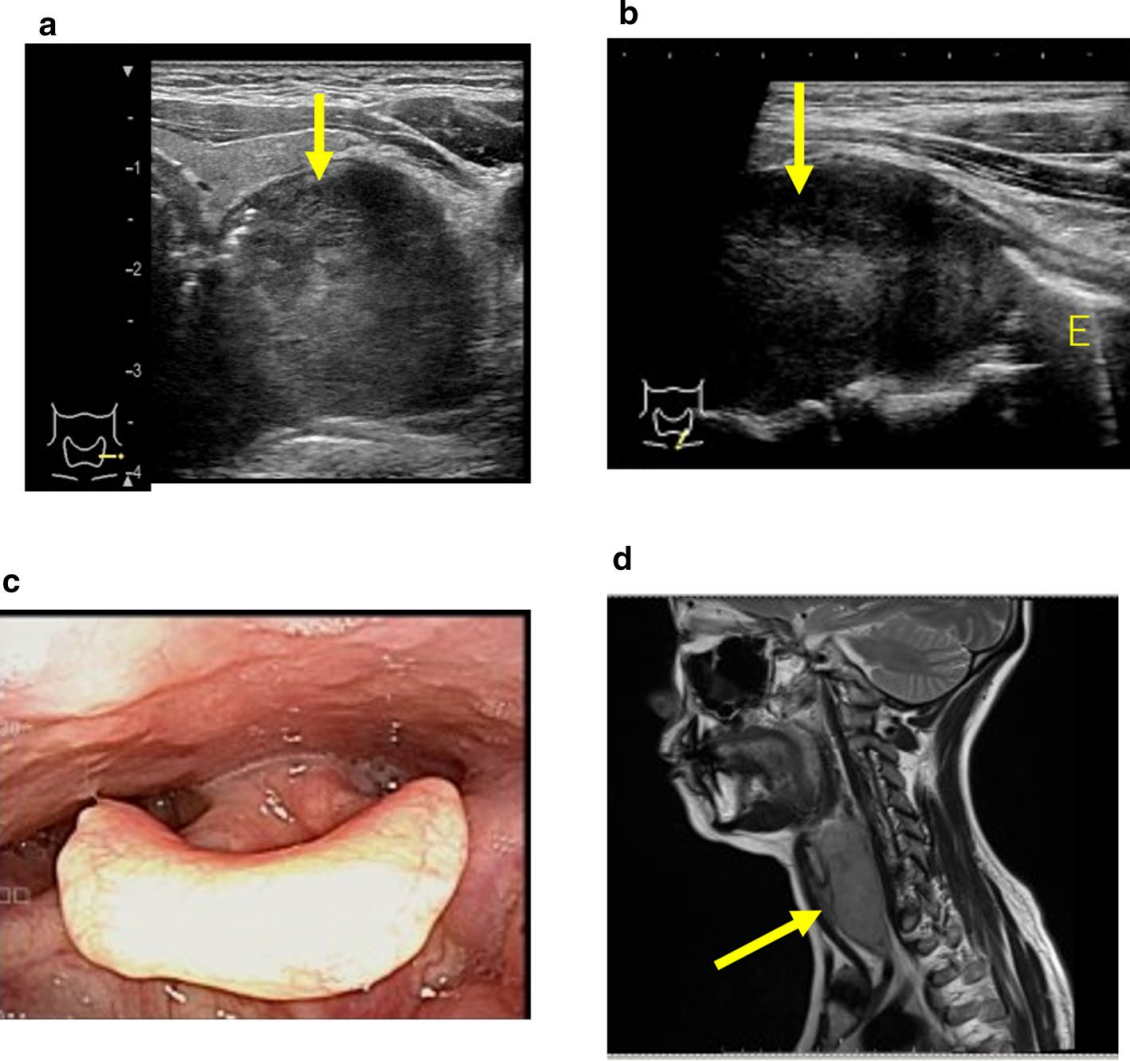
Advanced cancer of the cervical esophagus. a Transverse view of the lower part of the anterior neck: A tumor (↑) was found on the dorsal side of the left lobe of the thyroid gland. b Sagittal image of the lower part of the anterior neck: The tumor (↑) led to the cervical esophagus (E). c Laryngeal fiberscope findings: No obvious neoplastic lesions can be pointed out. d Cervical MRI findings (sagittal section): A tumor (↑) was found from the posterior hypopharyngeal ring to the cervical esophagus
